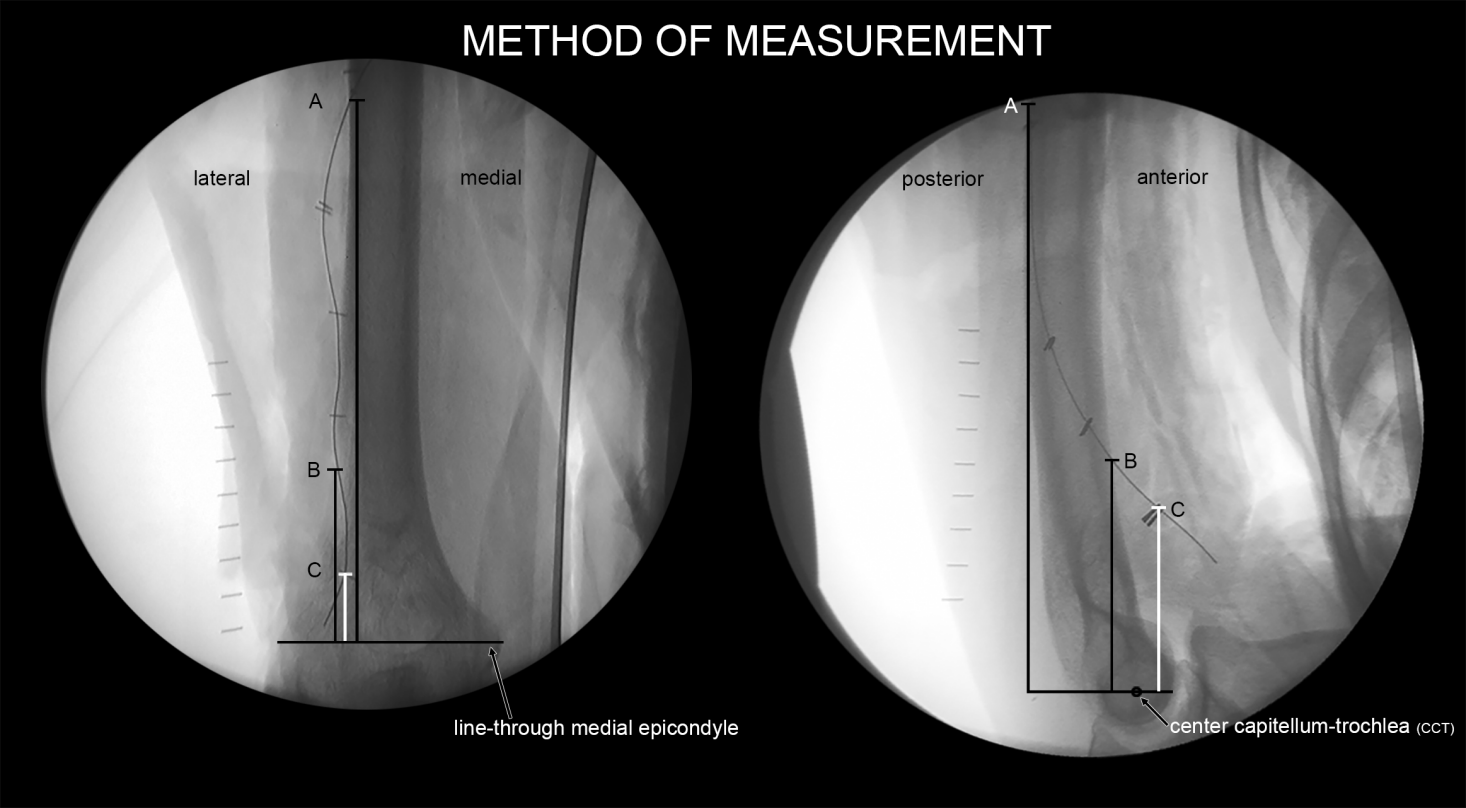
Radiographs (AP and lateral view) of prepared upper arm showing a marked radial nerve and method of measurements. Points of interest A, B and C are marked.
